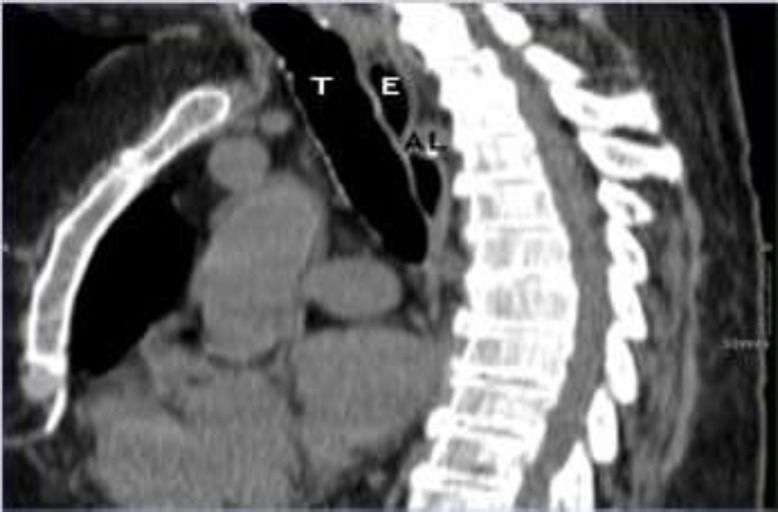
HRCT, sagittal section (T= trachea, E= esophagus, AL = arteria lusoria)
